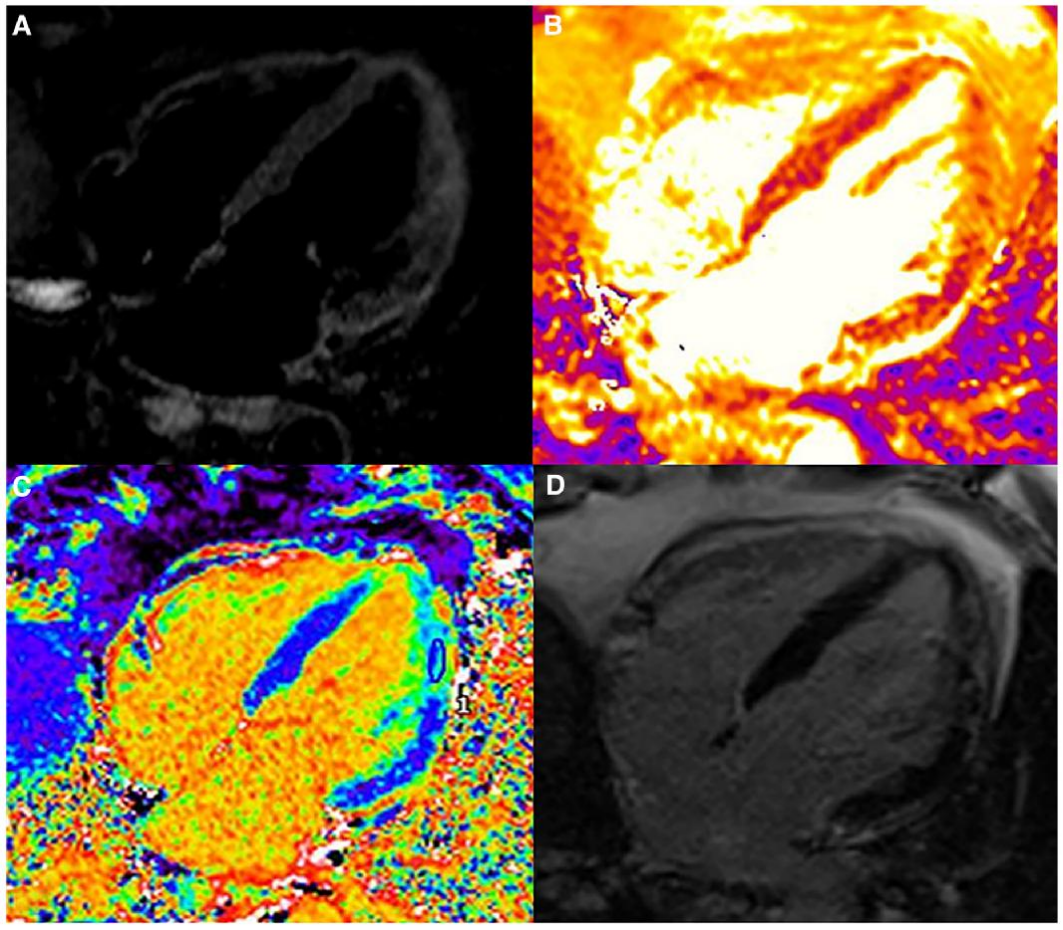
Cardiovascular MRI findings in myocarditis. Cardiovascular MRI in a patient with myocarditis showing high signal intensity on T2-weighted images in the anterolateral wall (STIR-T2, *A*) and increased T2 mapping values (70 ms, *B*) and ECV values (40%, *C*) in the same area. After injection of gadolinium contrast, enhancement with a non-ischaemic pattern involving the anterolateral wall is highlighted (*D*).
